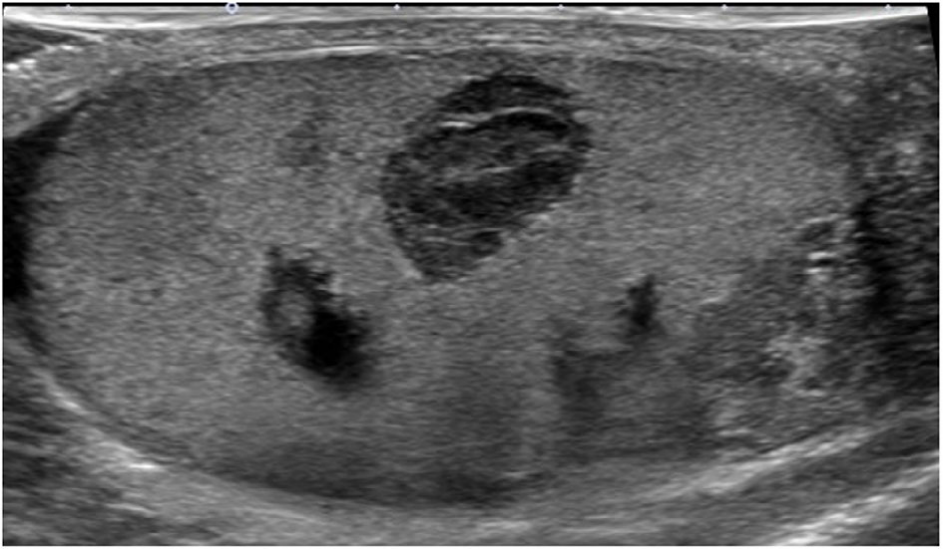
Ultrasonography image of the right testis on the first visit. Testis size was 47 × 21 × 22 mm. Several nodules with an unclear border were detected in the upper and lower poles.
